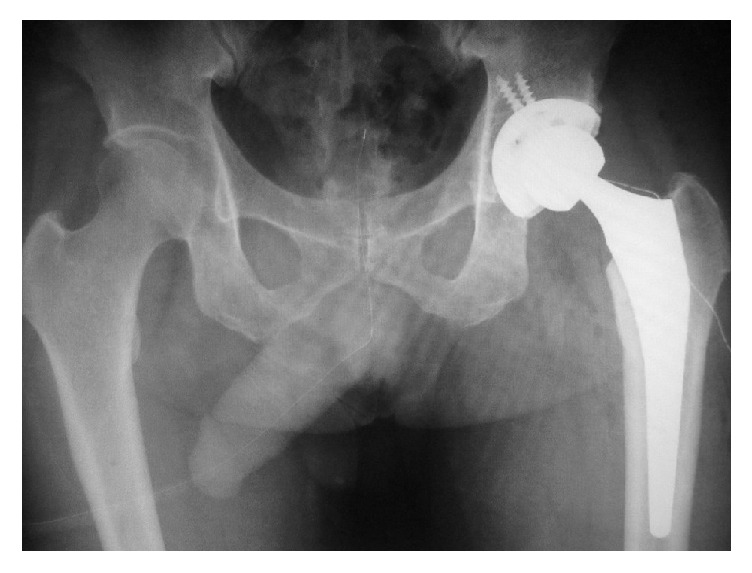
Radiographs of the hip joint after total hip arthroplasty.
